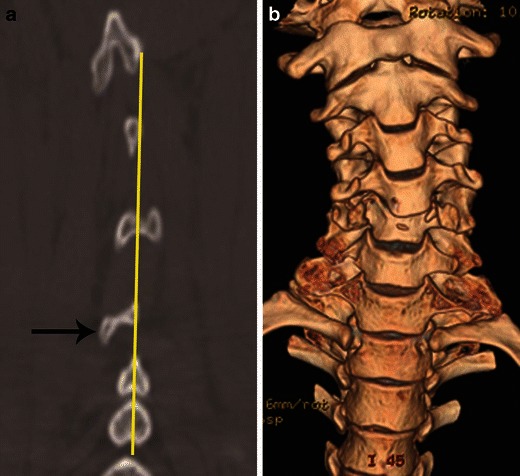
Rotational vector and deformity. a Posterior coronal CT image shows focal deviation of the C5 spinous process (*arrow*)—suggested by the interspinous line not passing through the rotated C5 spinous process. b Three-dimensional volume rendered coronal image shows rotational deformity of the C5/C6 vertebrae
